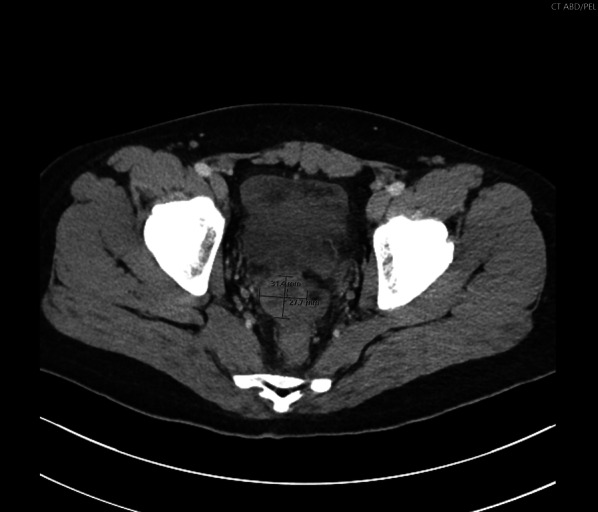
Transverse view of the abdominal CT demonstrating 31.4 × 27.7-cm appendiceal abscess by the appendiceal tip in the pelvis
